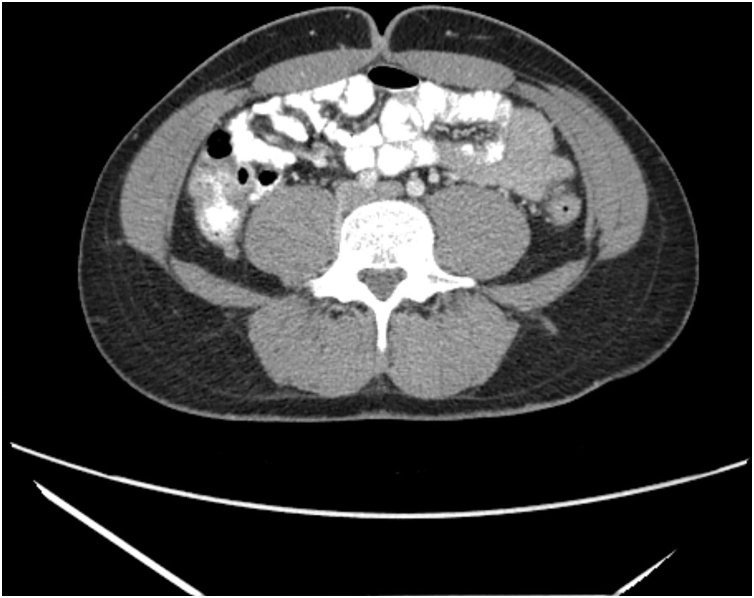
Axial view of CT abdomen and pelvis with PO contrast after 24h of non-operative management of SBO.
